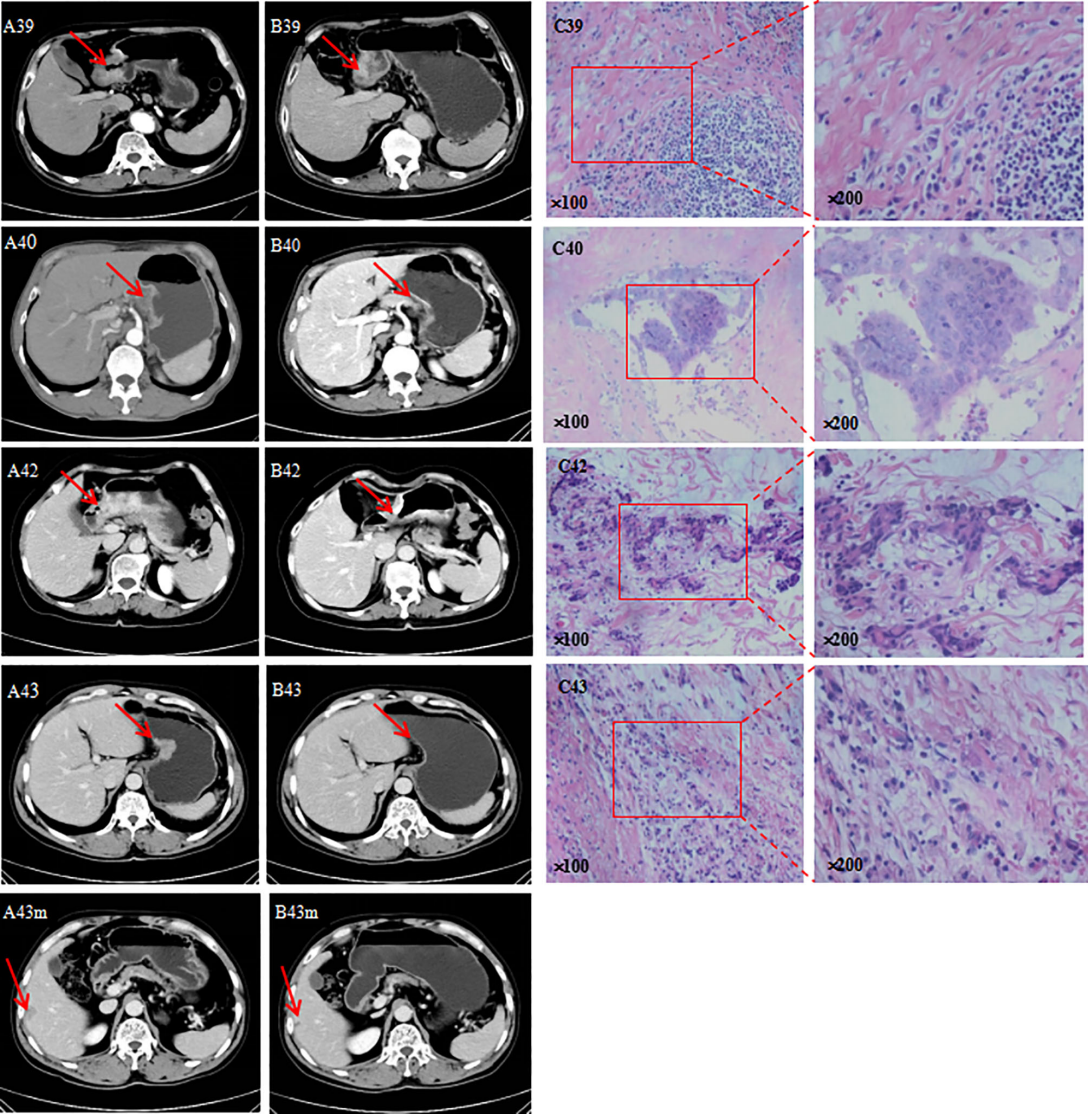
(A) CT before neoadjuvant chemotherapy; (B) CT after neoadjuvant chemotherapy; m, liver metastasis; (C) image pathology after neoadjuvant chemotherapy.
